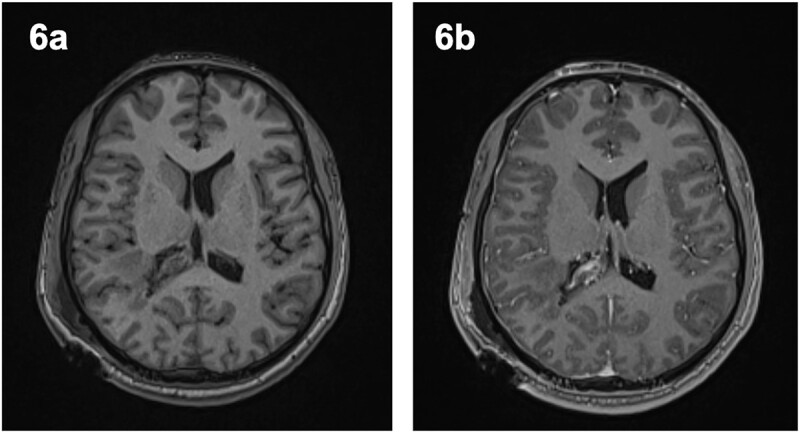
Post-operative magnetic resonance imaging revealed evidence of intraventricular enhancement, with no distinct lesion identified on axial T1-weighted imaging pre- (6a) and post-contrast (6b).
